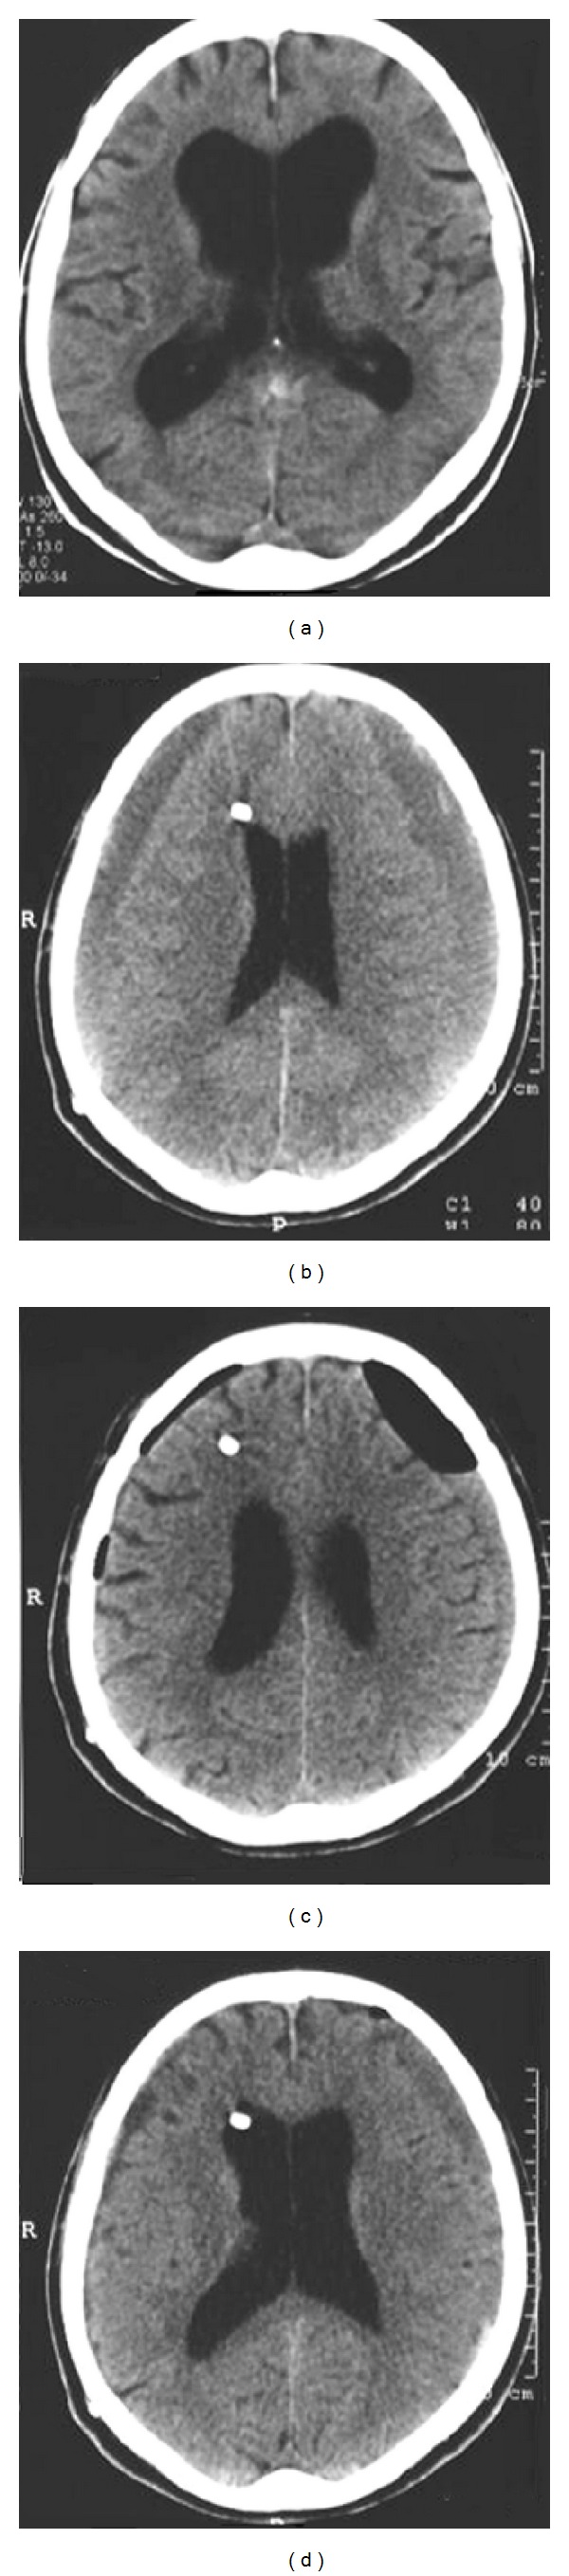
(a) CT scan showing a widened ventricular system. (b) CT scan 35 months after shunt implantation and bilateral traumatic chronic subdural hematomas were demonstrated. (c) Postoperative CT scan 2 days after surgical drainage and adjustment of the opening pressure. (d) CT scan two weeks after the adjustment. The hematomas were almost resolved.
